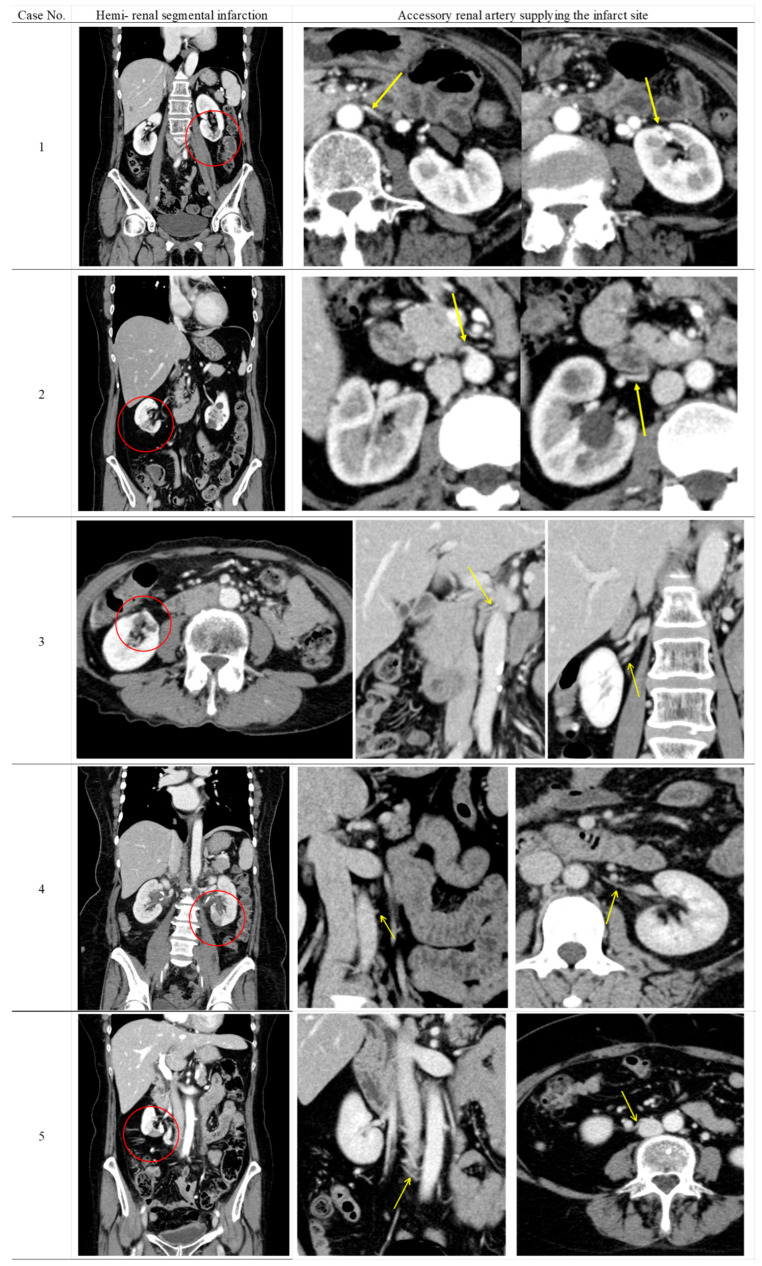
Contrast-enhanced computed tomography findings in five patients with renal perfusion defect potentially involving accessory renal artery obstruction.
